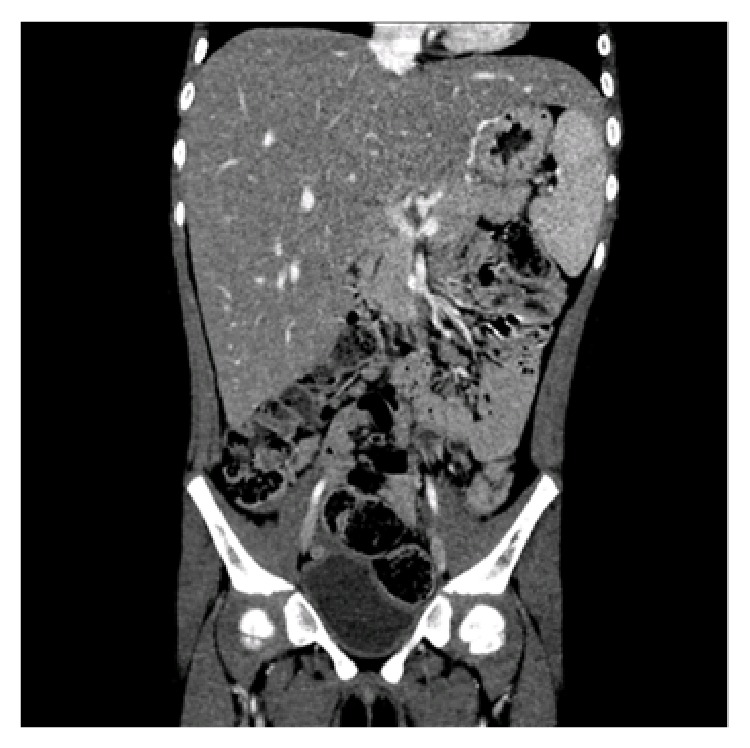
Computed tomography of abdomen and pelvis. The liver is enlarged, measuring up to 17 cm in width and craniocaudal dimension. The contour is smooth. The hepatic parenchyma is homogenous without a focal mass. There is no intrahepatic biliary dilatation. The portal and hepatic venous systems are patent and nondilated.
